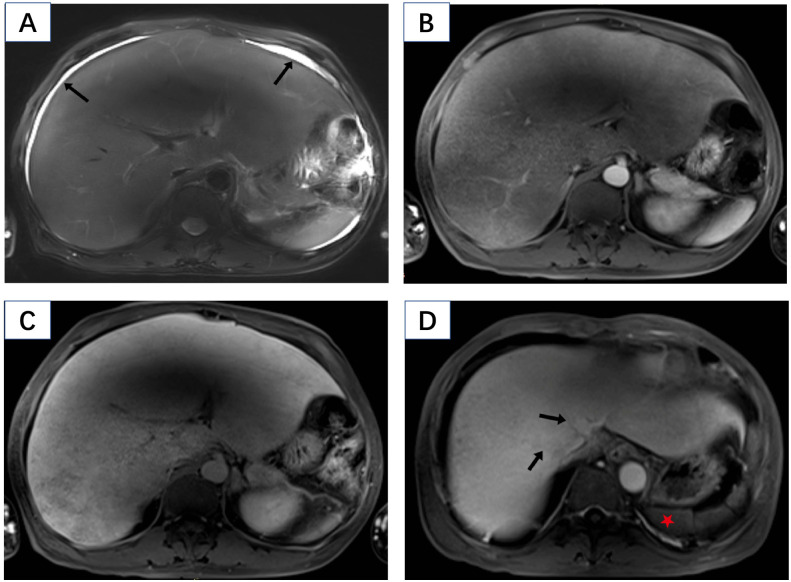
Hepatic AL amyloidosis in a 60-year-old male (A–C). (A) Axial T2-weighted MRI demonstrates hepatomegaly with finely textured hepatic parenchyma and perihepatic ascites (arrow). (B) Arterial phase of DCE-MRI shows mild enhancement of the hepatic parenchyma. (C) Equilibrium phase of DCE-MRI reveals delayed enhancement of the hepatic parenchyma. (D) Equilibrium phase of DCE-MRI in another 52-year-old male patient displays delayed parenchymal enhancement, with hepatic venous stenosis (arrow) and splenic hypoperfusion (red star).
